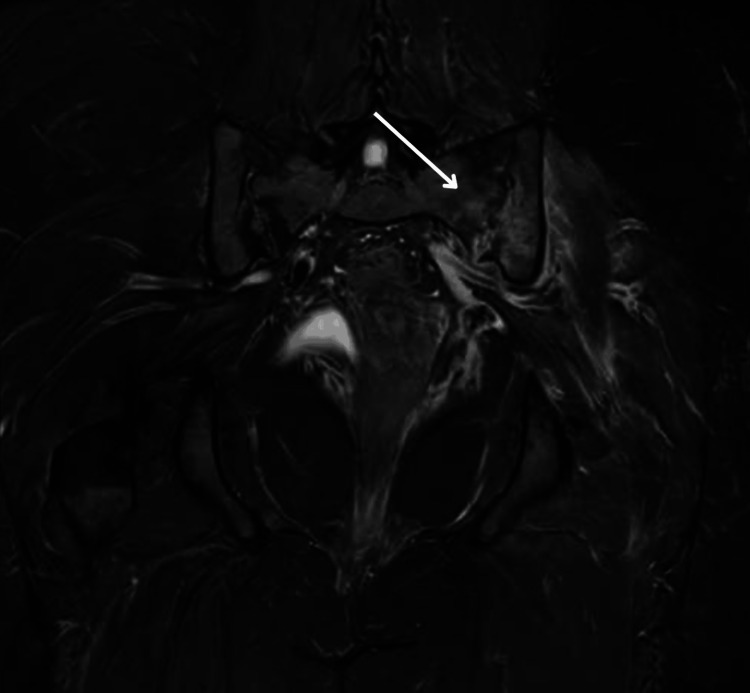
Coronal T2-weighted MRI of the pelvis Coronal T2-weighted sequence demonstrating increased signal intensity and periarticular edema involving the left sacroiliac joint (arrow), consistent with infectious sacroiliitis. The right sacroiliac joint appears preserved, highlighting the unilateral nature of the inflammatory process.
